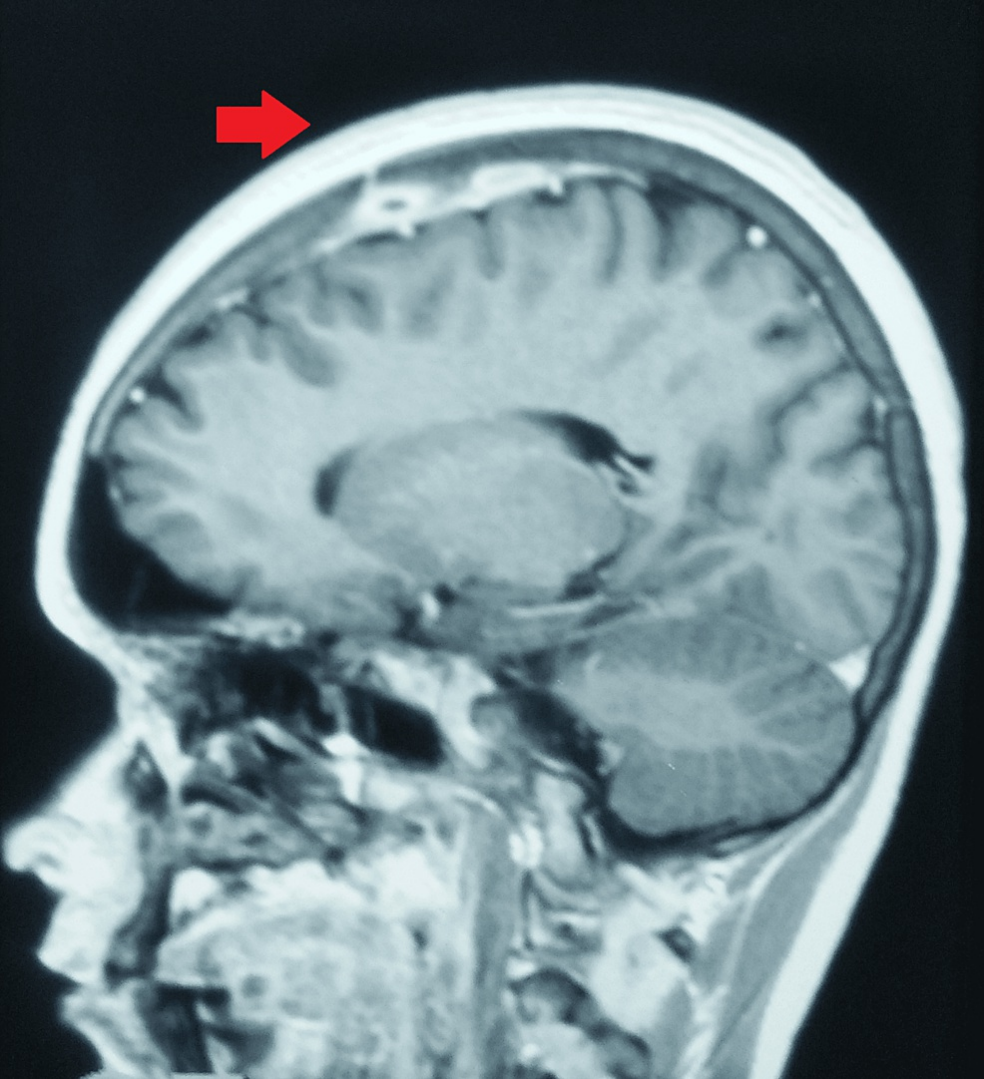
MRI showing a well-defined lesion situated on the left parietal bone MRI: Magnetic resonance imaging
